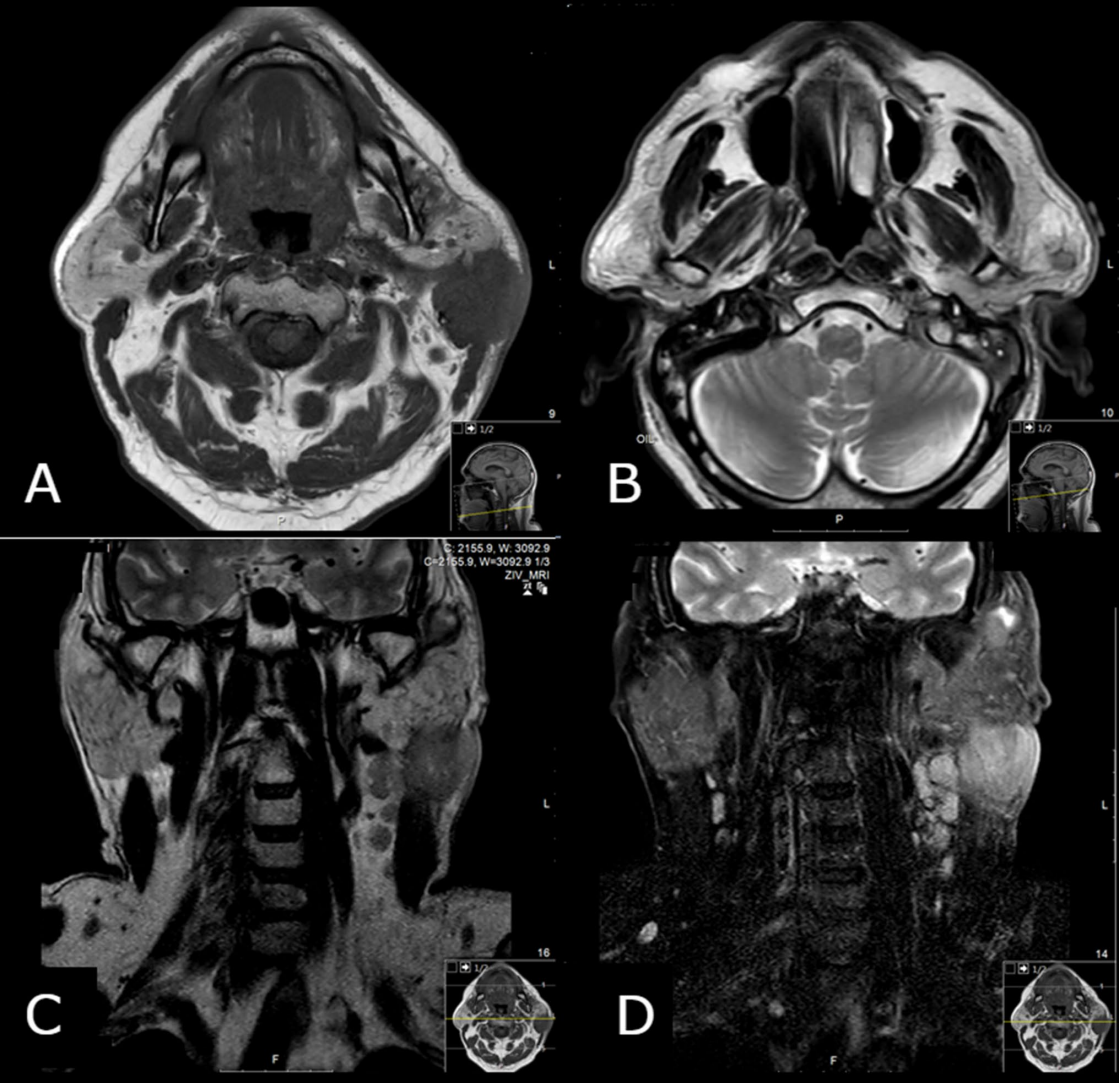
A MRI T1 lobulated, irregular mass, located in the posteroinferior segment of the superficial segment of the superficial lobe in the left parotid gland. B MRI T2 with areas of extensive central necrosis, septation. C Several suspected lymph nodule in the left infraparotid area and level II and III. D Peripheral wall enhancement
